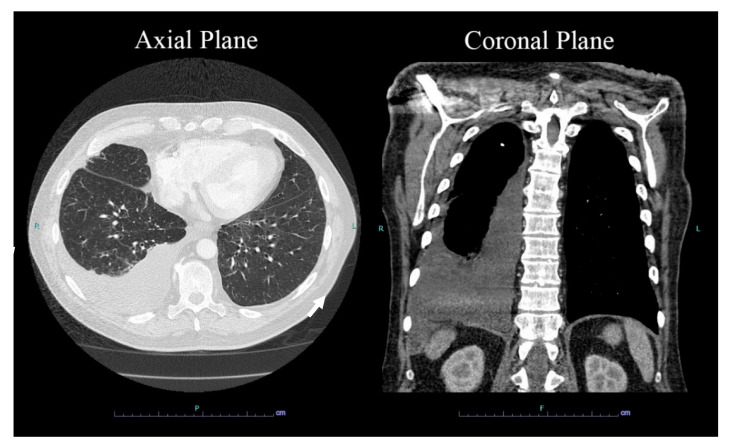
Imaging studies. Axial plane (left panel) and coronal plane (right panel) computed tomography (CT) scan with IV contrast of the chest showing moderate right pleural effusion (white arrows) with a small amount of compressive airspace disease at the right base.
